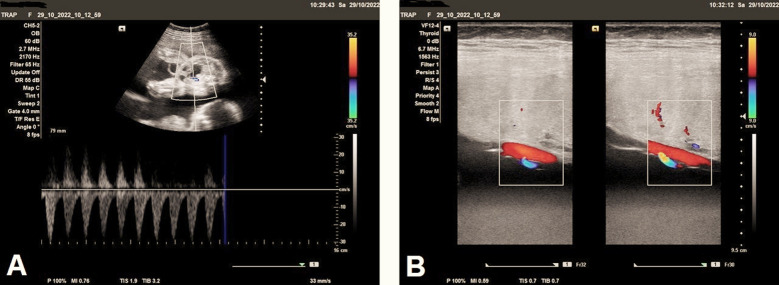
(A) Color and spectral Doppler examination shows acardiac twin with reversed direction of vascularity seen in iliac blood vessels. (B) Color and spectral Doppler examination shows anastomotic superficial blood vessels seen crossing the placental surface connecting the short umbilical cord vasculature of the acardiac twin to the pump twin.
